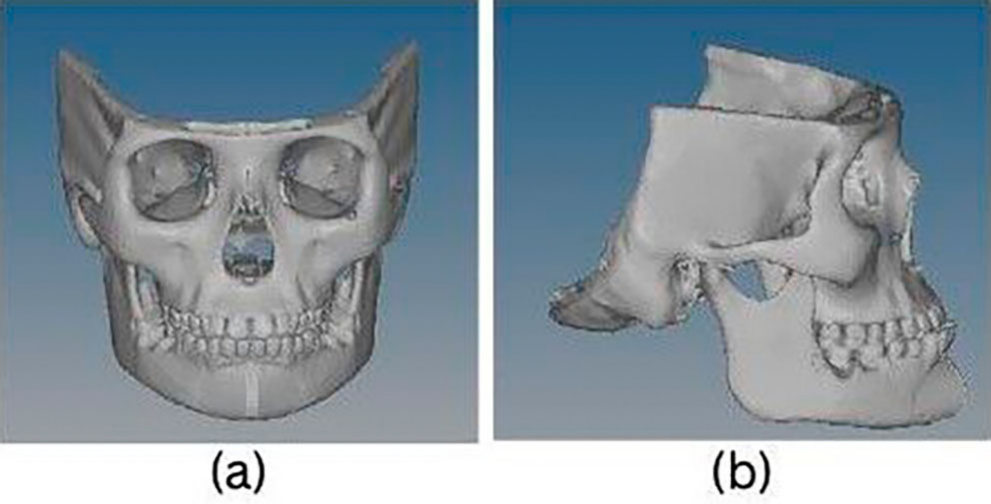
(a and b): CT scan of a skull with Class III malocclusion seen in (a) frontal view and (b) lateral view.
